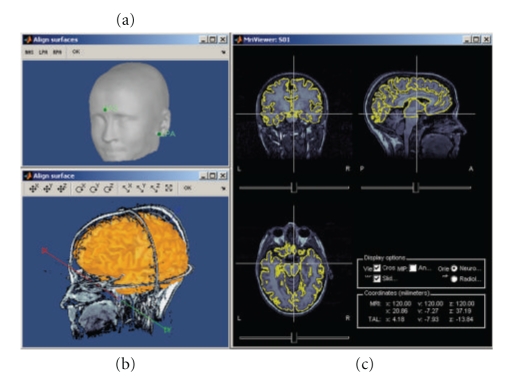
Registration of MRI data volumes with corresponding surface meshes.
